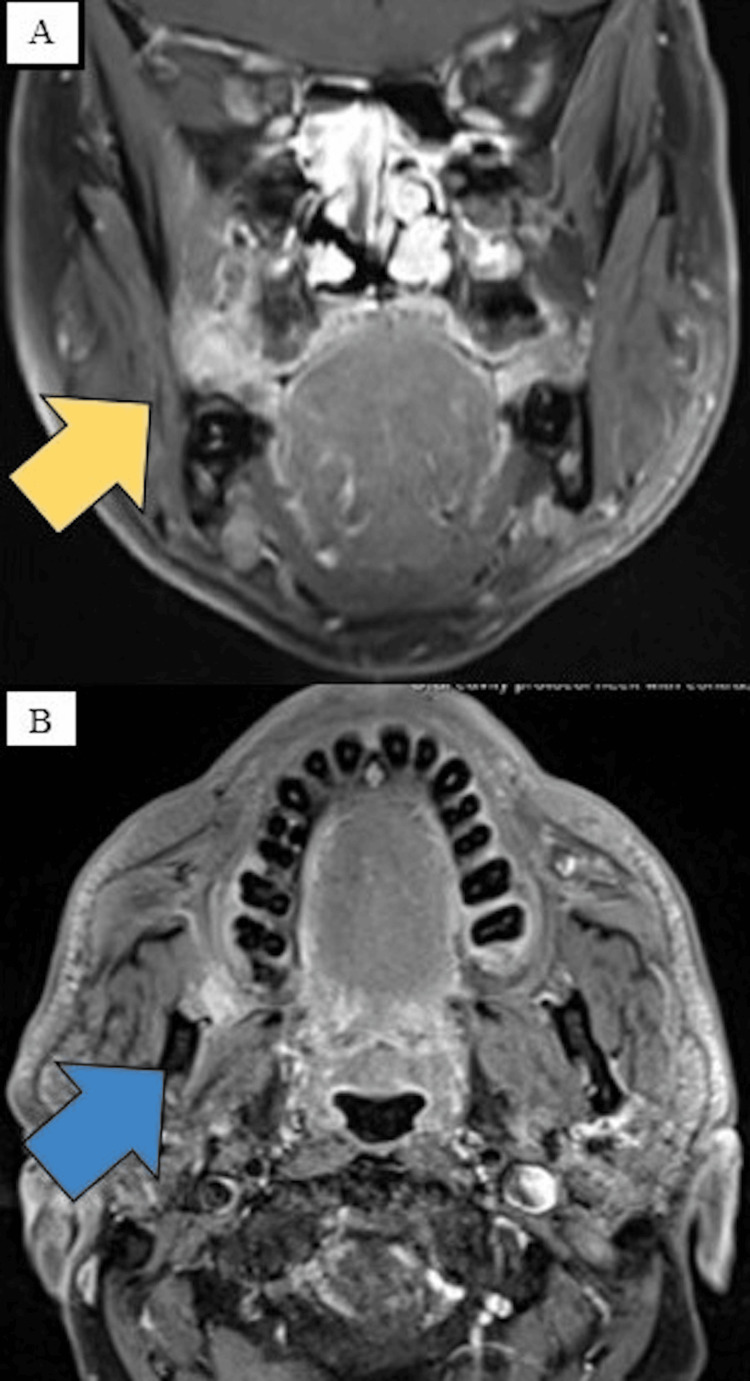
(A) Contrast-enhanced MRI imaging shows a soft tissue lesion in the right retromolar extending superiorly to the level of the maxillary alveolus (yellow arrow). No destruction of the underlying bone was observed. The lesion measurement was about 1.5 x 0.9 x 1.6 cm. (B) The soft tissue enhancement reaches the anterior inferior aspect of the lateral pterygoid muscle (blue arrow).
